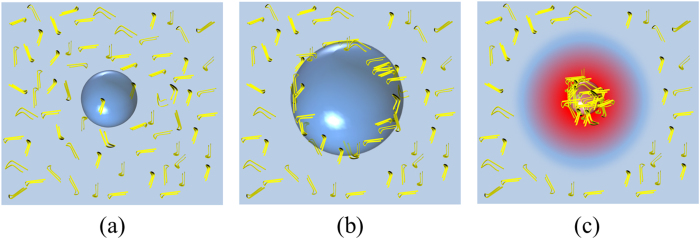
Aggregation acceleration model focused on cavitation-bubble dynamics. (a) Bubble is generated in solution by negative pressure of ultrasonic wave. (b) Bubble grows in the negative-pressure phase, during which A*β* monomers (yellow hairpin-shaped bars) are absorbed on bubble surface by hydrophobic interaction. (c) Bubble collapses at positive pressure of ultrasonic wave, leading to local condensation of A*β* monomers and temperature increase in and near the bubble.
